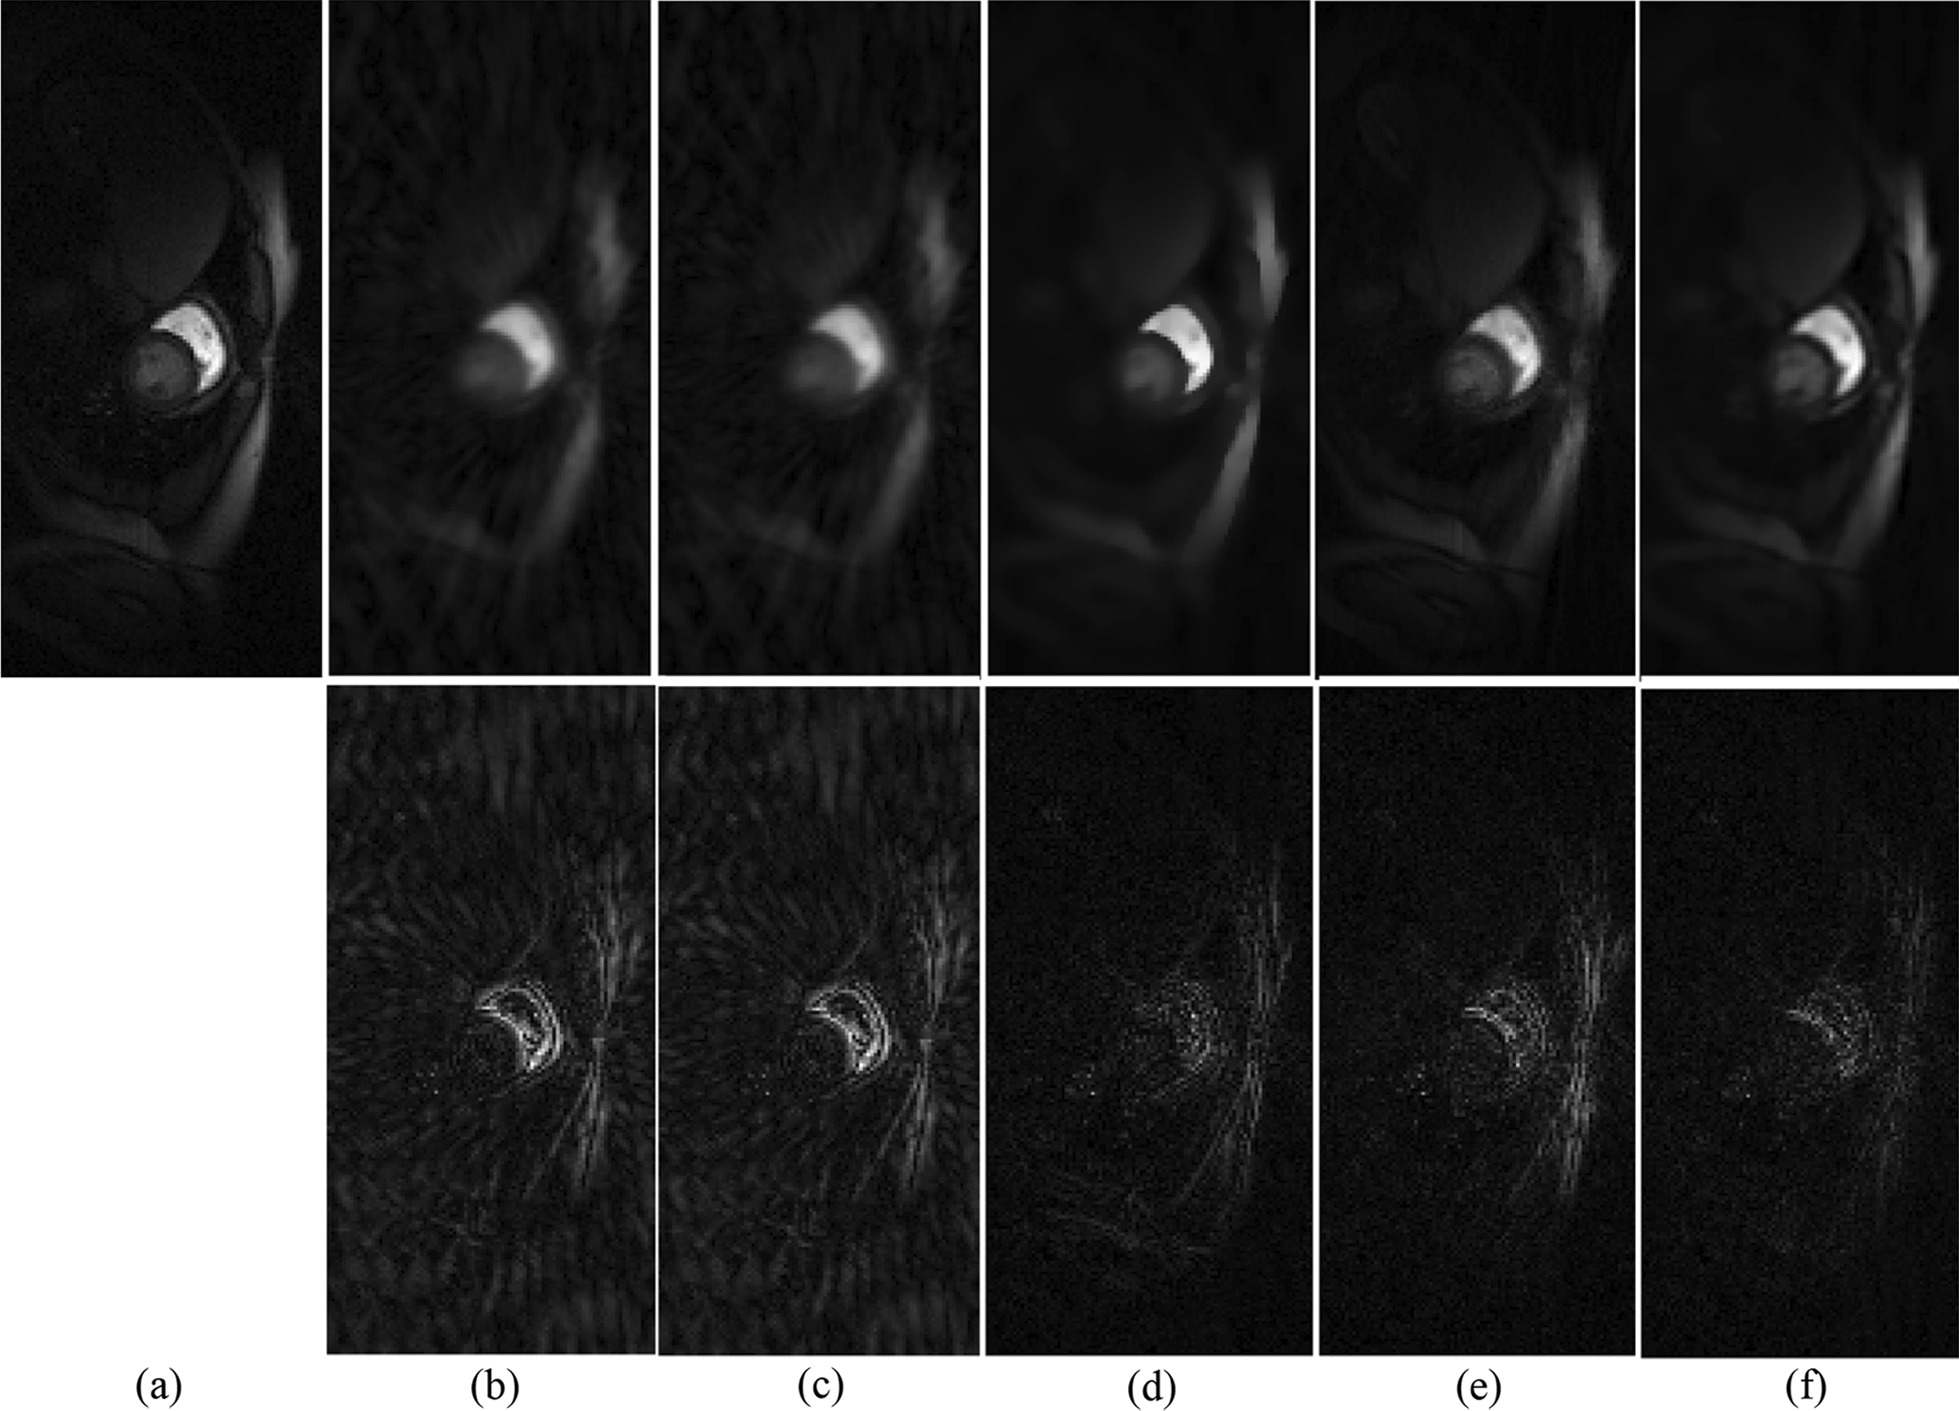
Performance evaluation of *k-t* TGV-TD method in comparison with HOSVD, *k-t* SLR, 3D-TGV, and L + S methods on the cardiac perfusion dataset. The first row is the reconstructed MR image with acceleration factor 6; the second row is the error map. a MRI reconstructed with fully sampled k space data; b–f reconstructed MRI and the corresponding error images by using HOSVD, *k-t* SLR, 3D-TGV, L + S, and *k-t* TGV-TD respectively
